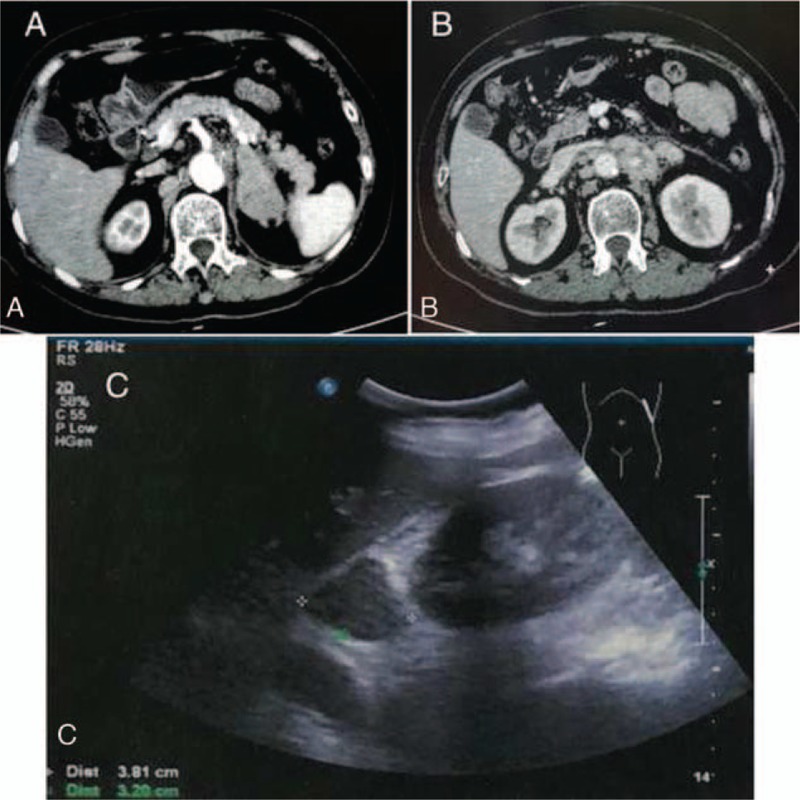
(A) Enhanced CT scan showing a 5.1 × 3.0 cm, uneven enhanced, rounded mass in the left adrenal gland. (B) Enhanced CT scan showing enlarged lymph nodes in the abdominal cavity and behind peritoneum. (C) Ultrasonography showed a 3.8 × 3.4 × 3.2 cm mass on the left adrenal gland.
